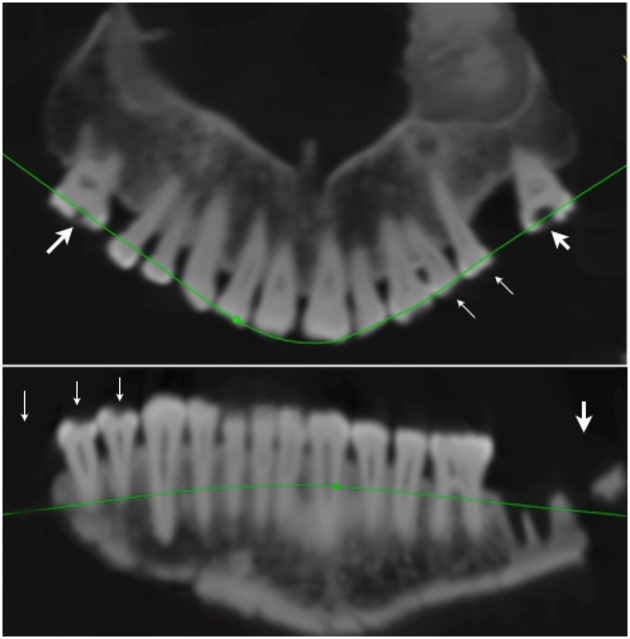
CT based panoramic radiograph of the maxillary (top) and mandibular dentition (bottom). Two teeth have been extracted before radiographic examination (right first and left second mandibular molar), while the left mandibular wisdom tooth, whose clinical crown was completely destroyed, was not in its alveolus, and both maxillary first molars have been lost intra vitam. Radiographically visible deep carious lesions are shown by large arrows; small carious lesions that are only visible macroscopically are shown by small arrows.
